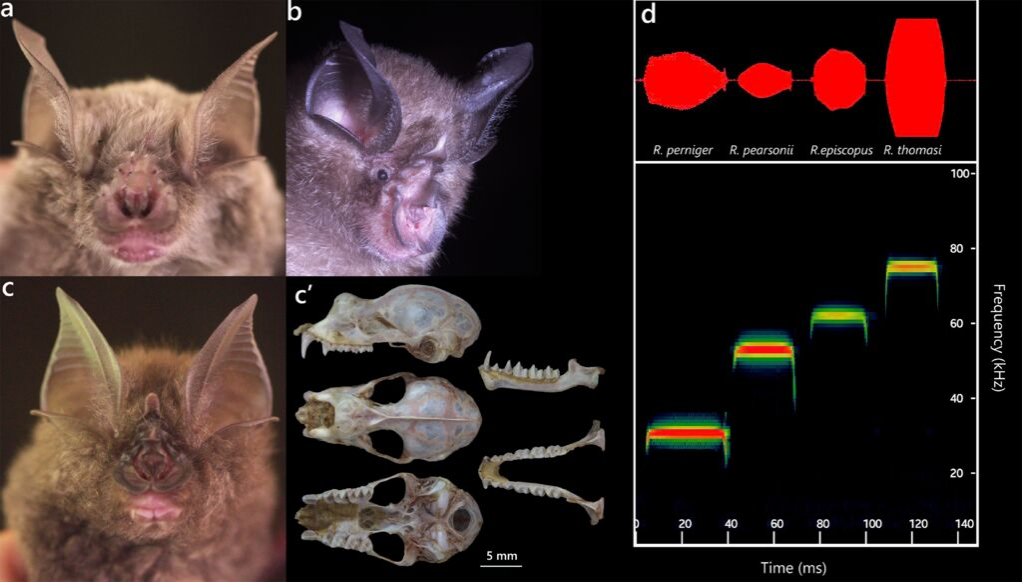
Selected Rhinolophydae species recorded: (a) *R*.* thomasi*; (b) *R*.* pusillus*; (c) *R*.* pearsonii*; (c’) Five aspects of the cranium and mandible of *R*.* pearsonii*; (d) Ultrasonic echolocation calls of Rhinolophids.
